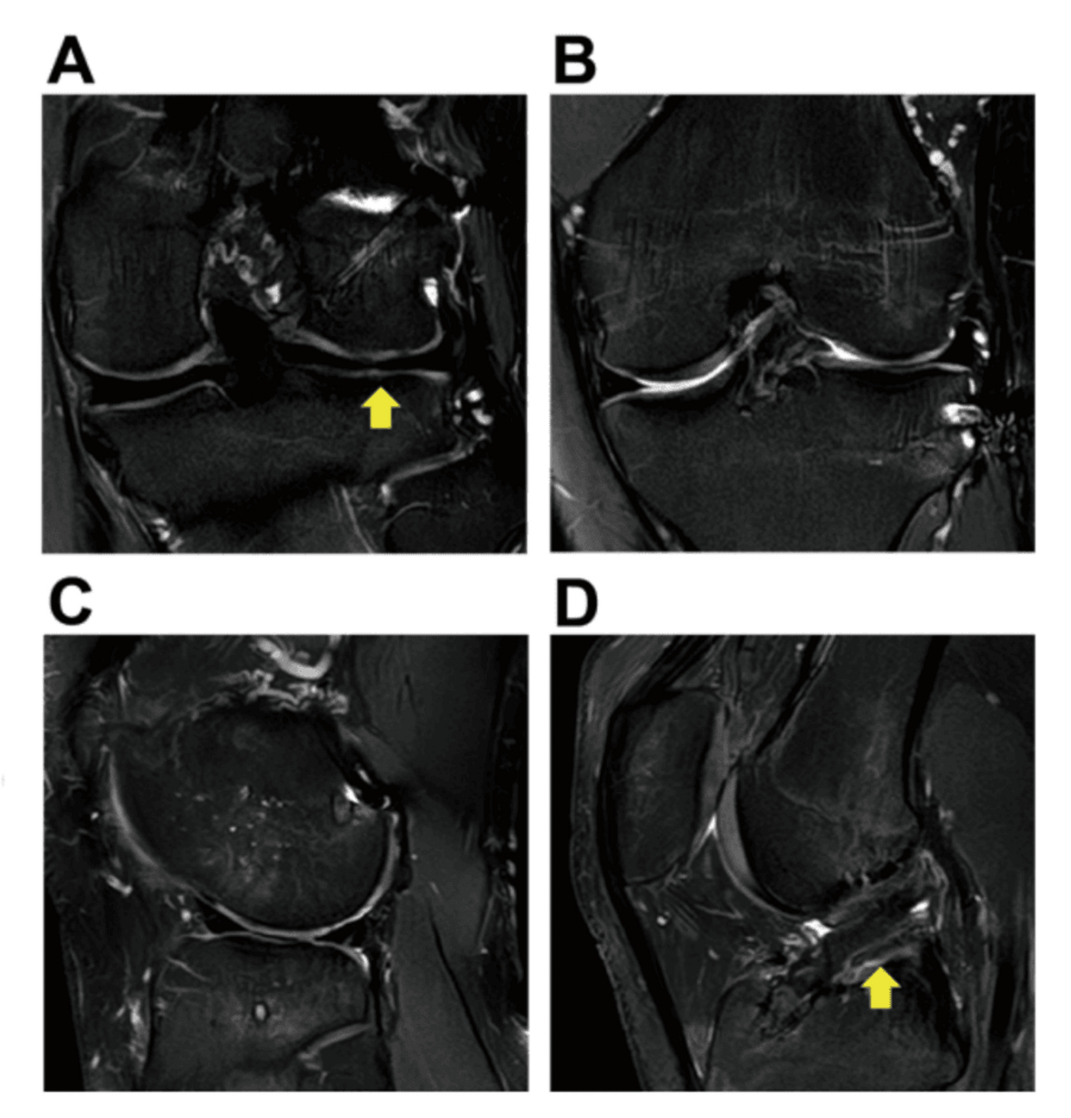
MR images two years after ACL reconstruction. (A) A coronal view slice showing the well-repaired posterior segment of the lateral meniscus (yellow arrow). (B) A coronal view slice showing the slightly extruded middle segment of the lateral meniscus adjacent to the fixed fracture site. (C) A sagittal view slice showing no obvious posterior extrusion of the lateral meniscus. (D) A sagittal view slice showing the reconstructed ACL (yellow arrow). MR: magnetic resonance; ACL: anterior cruciate ligament
